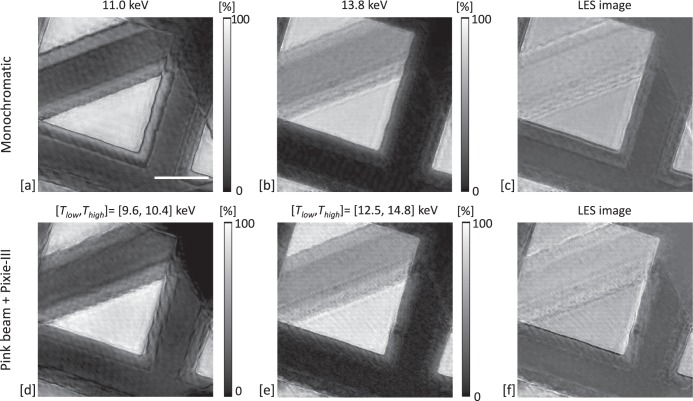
Top row: reference reconstructed images (modulus of the complex-valued ptychographs) acquired with the detector in energy integration mode and by using monochromatic beams at 11.0 keV (a) and 13.8 keV (b). The digital subtraction of (a,b) is reported in (c). Bottom row: reconstructed images acquired by using pink beam and the detector in colour mode when considering [*T*_*low*_, *T*_*high*_] = [9.6, 10.4] keV (d) and [*T*_*low*_, *T*_*high*_] = [12.5, 14.8] keV (e). The digital subtraction of (d,e) is reported in (f). [Scale bar = 25.0 m. Color bar for the above and below images representing the X-ray transmission].
